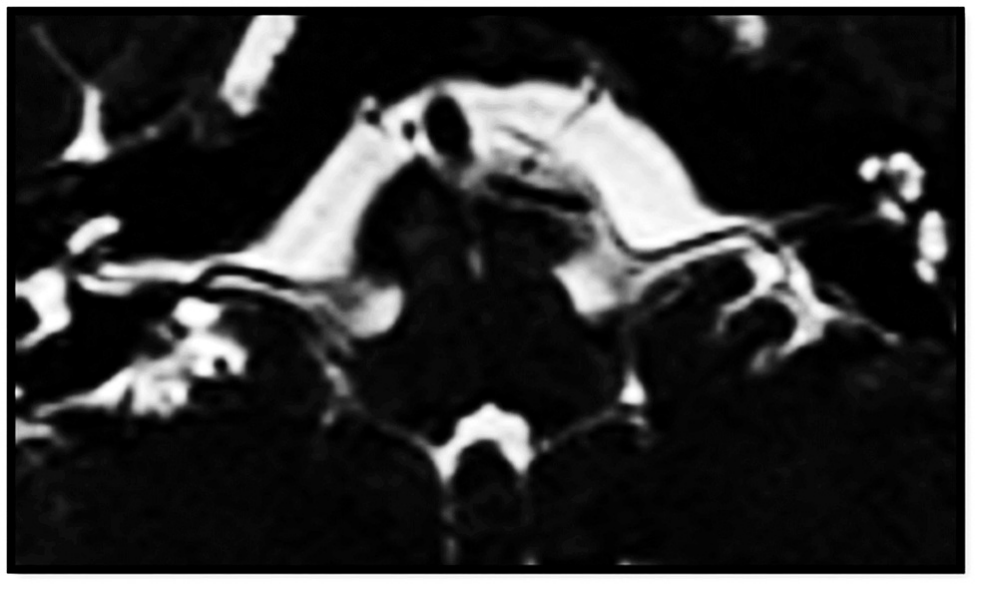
Chavda type I vascular loop. Axial high-resolution T2-weighted 3D-CISS sequence demonstrates bilateral type I Chavda vascular loop in the CPA cisterns. AICA vascular loop borders the internal auditory meatus without entering the IAC. CISS = constructive interference in steady state; CPA = cerebellopontine angle; AICA = anterior inferior cerebellar artery; IAC = internal auditory canal
